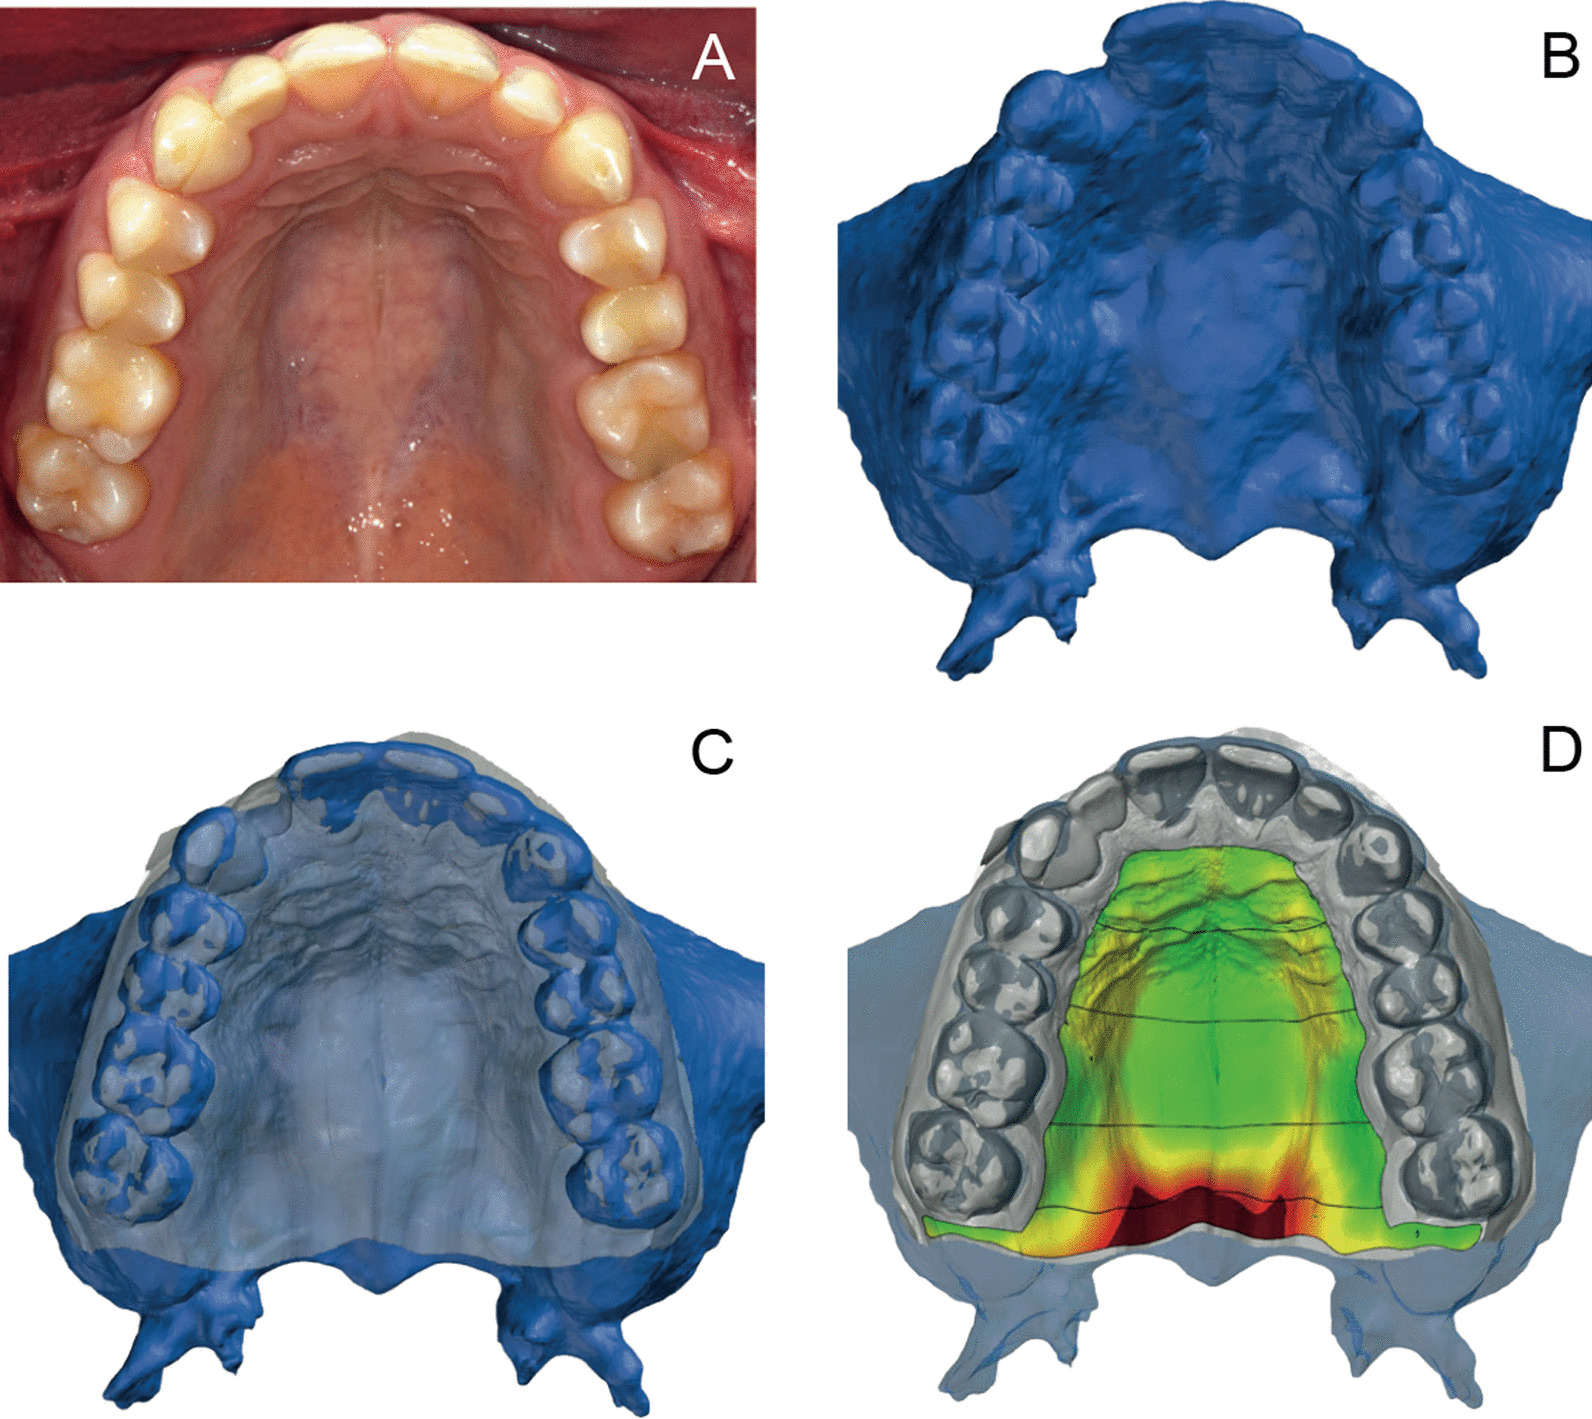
Virtual models merged to evaluate the volume of palatal soft tissues. A Clinical situation; B CBCT bone model; C merged CBCT and intraoral surface model; D colour-coded distance map representing the deviation between the two model surfaces at defined regions of interest (areas between the horizontal lines). Colour coding ranges from green (contacting model surfaces) to dark red (model surfaces separated by a large distance)
